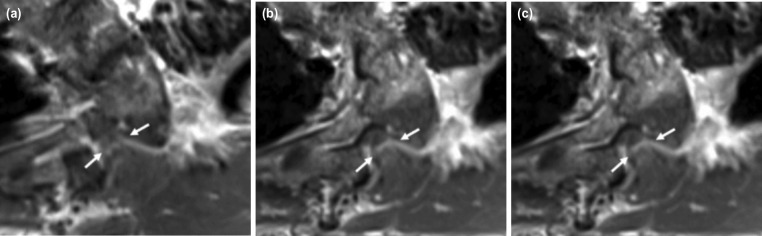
(a, b, c) MRI of the spine of Case 4 showing cord compression at T4/5 level due to a neurofibroma (white arrow).
